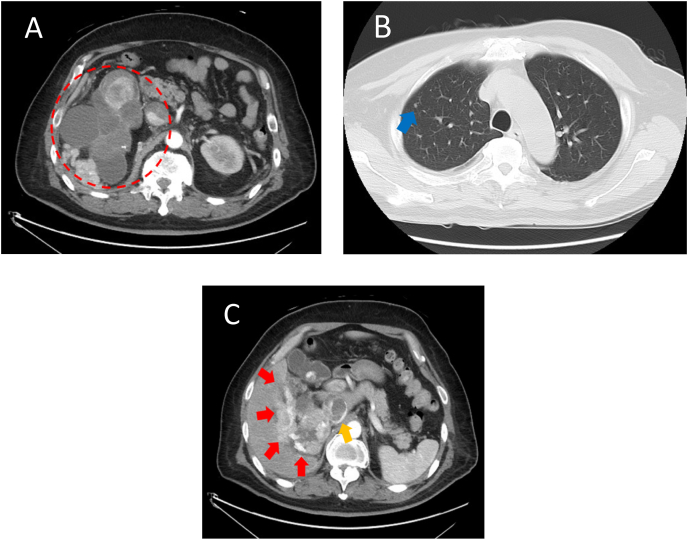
Computed tomography reveals a hypervascular tumor accompanied by a cystic lesion with a maximum diameter of 16 cm in the right kidney (A), with lung metastasis (B) and invasion into the inferior vena cava, liver, and adrenal gland (C).
